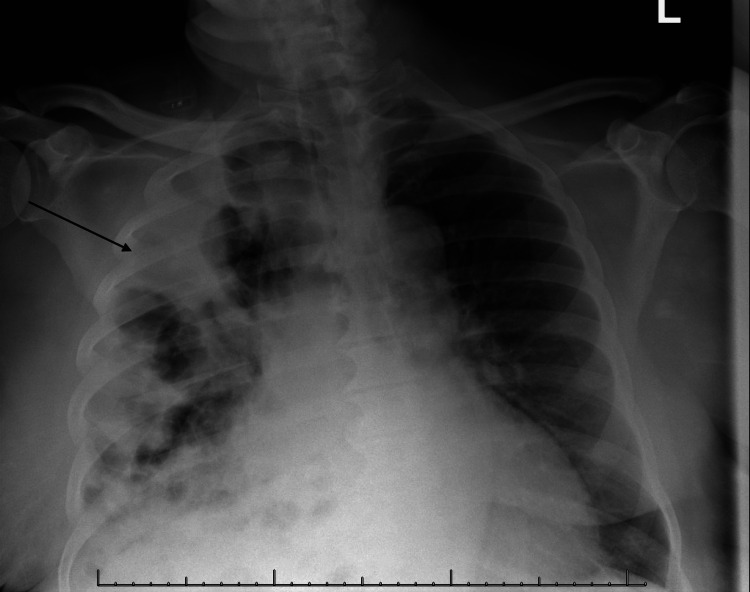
Chest X-ray showing multilobar opacities in the right lung fields (arrow)
